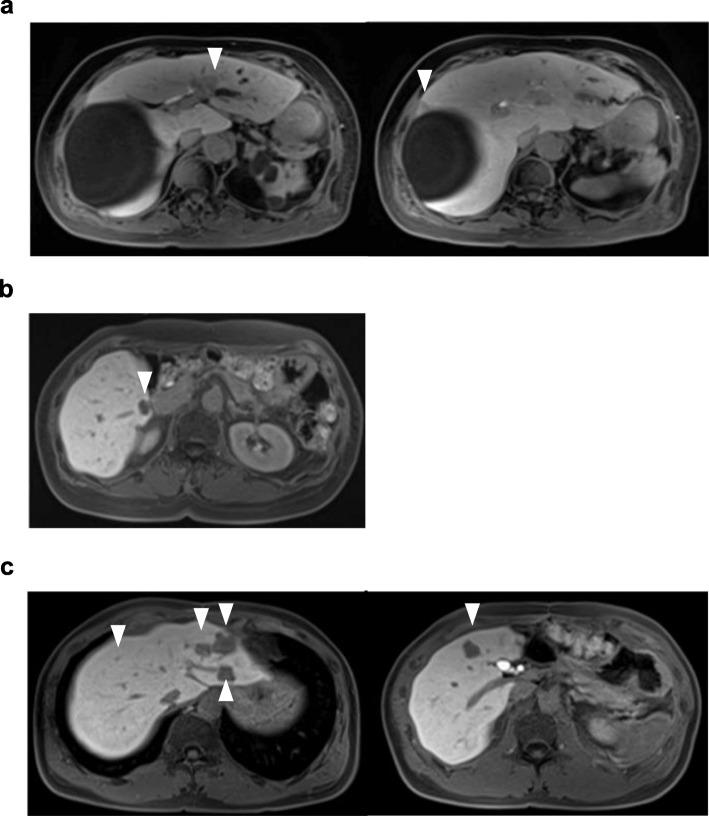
The locations of liver metastases on MRI in the hepatocyte phase. The locations of the tumors are shown with the arrowhead. a Case 1. Liver metastases were detected in segments 2 and 5. b Case 2. Liver metastases were detected in segment 6. c Case 3. Liver metastases were detected in segments 2, 3, 5, and 8. MRI, magnetic resonance imaging
